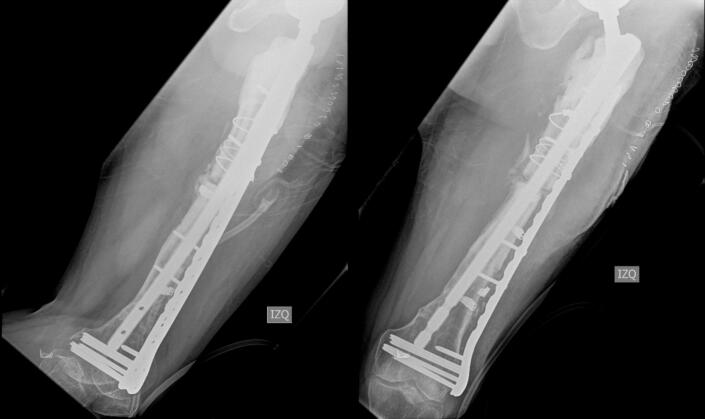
Postoperative AP and lateral radiographs of the femur showing integration and stability of the distal alloprosthetic complex.
